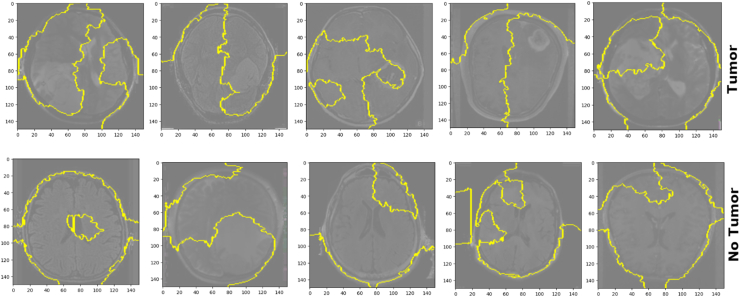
LIME explanations for MRI images of brain tumors. The top row shows images of tumors, whereas the bottom row shows images without tumors. The yellow outlines highlight the areas that most influenced the model's decision.
